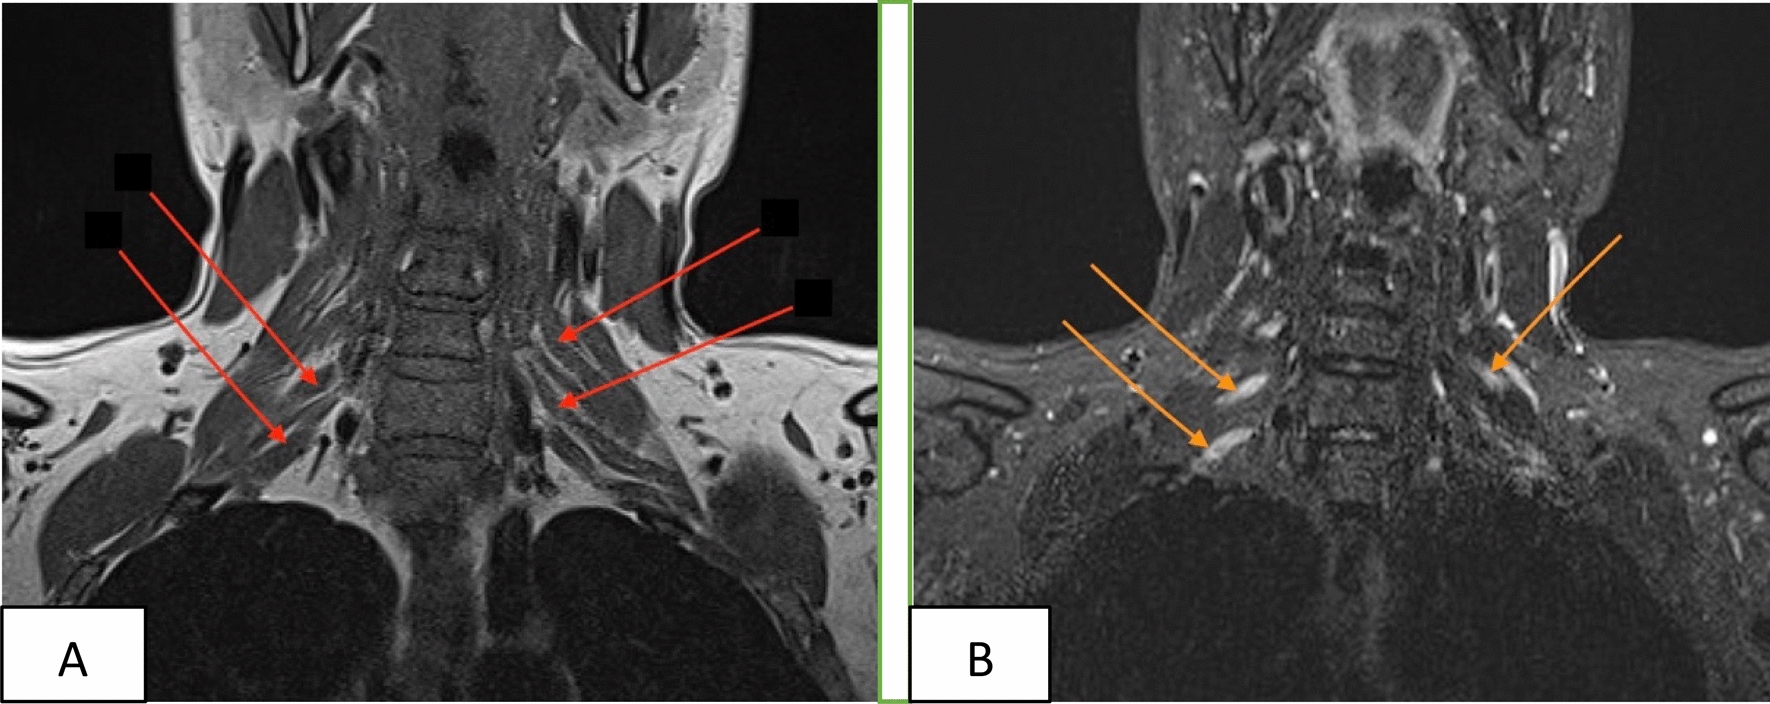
Patient’s brachial plexus magnetic resonance imaging. A Bilateral brachial plexus hypertrophy on T1 sequence (red arrows). B Bilateral brachial plexus hyperintensity, more marked on the right, on short tau inversion recovery sequences (orange arrows)
